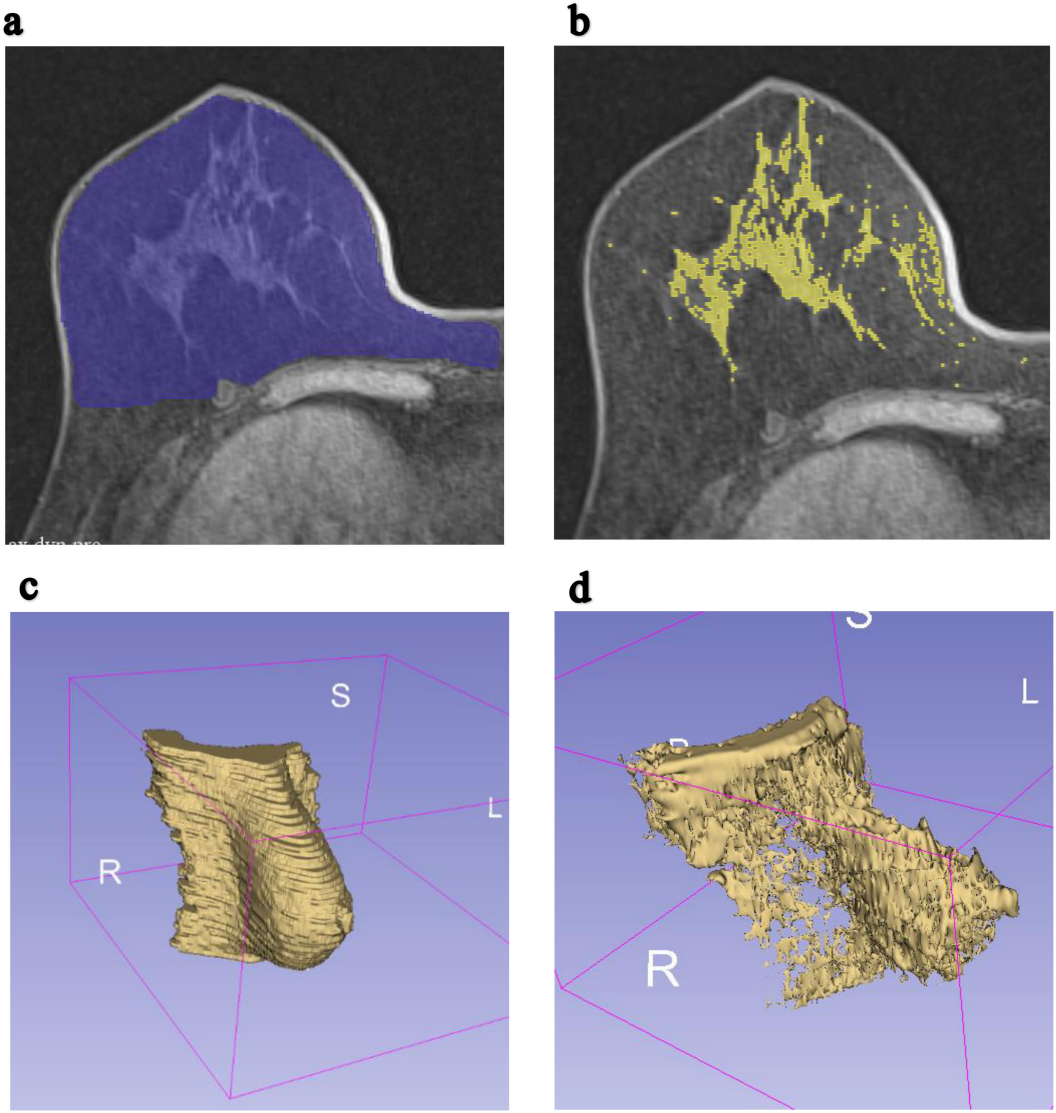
Example of MRI T1-weighted breast axial images were segmented to compute total breast volume and fibroglandular volume. (a) ROI for a single slice of the right breast in the axial plane. (b) ROI for a single slice of the fibroglandular tissue of the right breast in the axial plane. (c) The constructed 3D image of total volume of the contralateral breast. (d) The constructed 3D image of fibroglandular volume of the contralateral breast.
